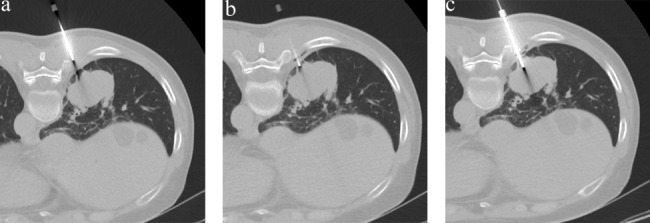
Axial CT images of a 56-year-old woman with a mass in the right lower lobe, who received pleural anesthesia. a. The coaxial needle entered the extra-thoracic muscle according to the given direction and angle. b. After injecting a small amount of anesthetic, CT was taken to confirm the position. Accumulation of anesthetic and incidental air bubbles is noted in the extra-pleural space. c. The coaxial introducer passed through the pleural and was further advanced to place the tip in the target lesion. The patient complained of mild pain (pain score 2).
